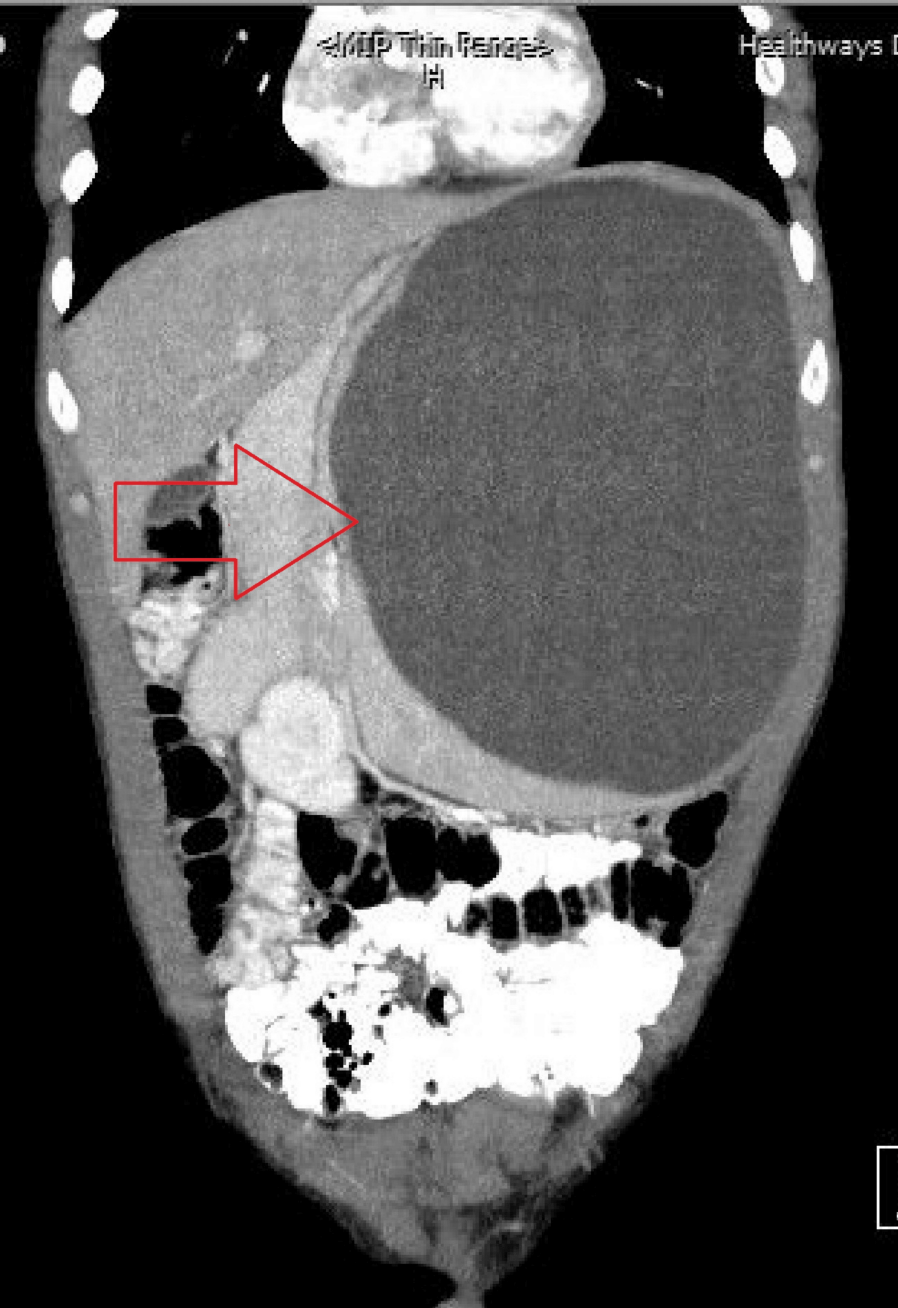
CT scan of the patient (anteroposterior view) Arrow showing splenic cyst on CT scan (anteroposterior (AP) view).
